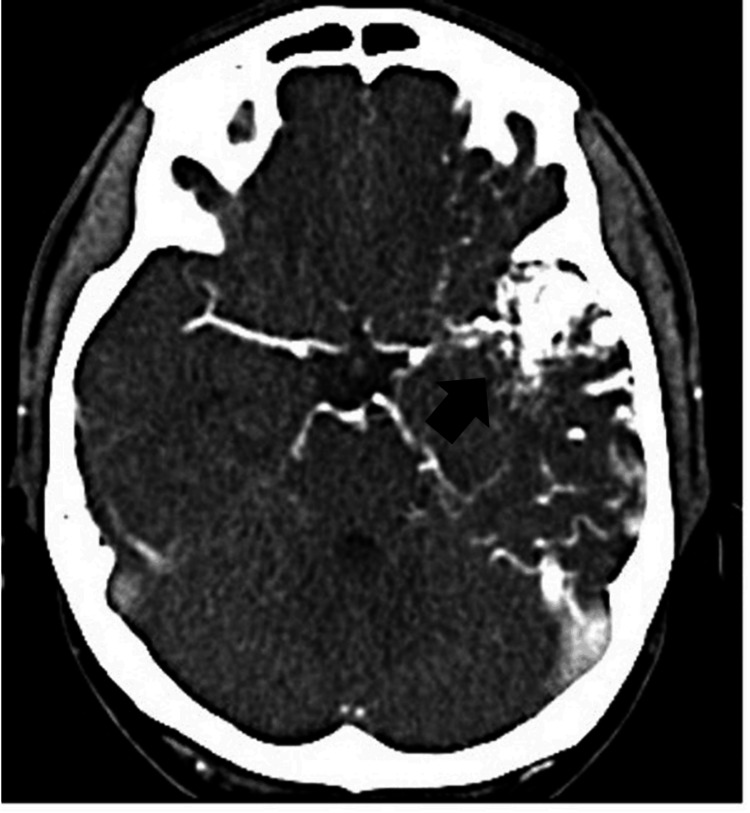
Contrast-enhanced computed tomography showing a cerebral arteriovenous malformation (black arrow) with a maximum diameter of 32 mm within the left temporal lobe.
